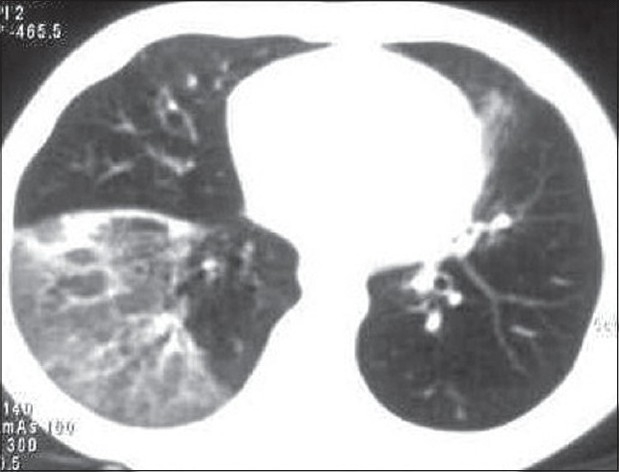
CT chest at the end of 10 weeks showing partially resolved pneumonia bilaterally and features of bronchiolitis obliterans (terminal bronchiolar dilatation with areas of air trapping).
